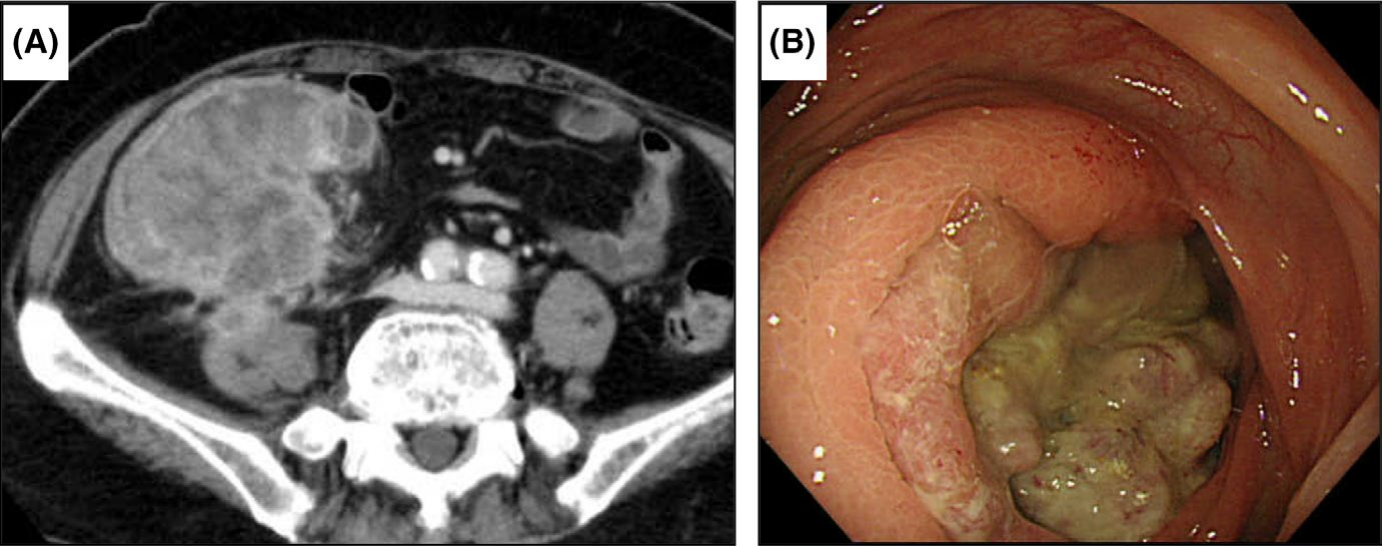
(A) Computed tomography scan showed a tumor, measuring 9 × 8 cm, in the ascending colon, suspectedly infiltrating the psoas major muscle. (B) Colonoscopy revealed a circumferential tumor in the ascending colon
